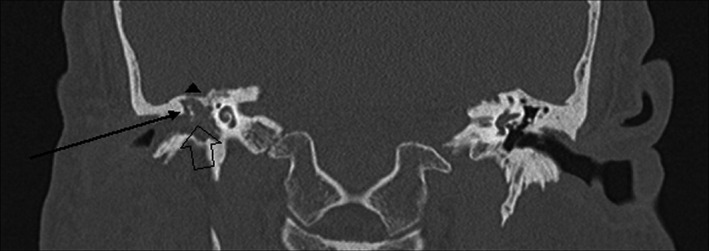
Coronal temporal bone CT view of complete right middle ear and external auditory canal opacification along with evidence of scutum erosion (line arrow), thinning of tegmen tympani (below triangle), and ossicular erosion (arrow)
